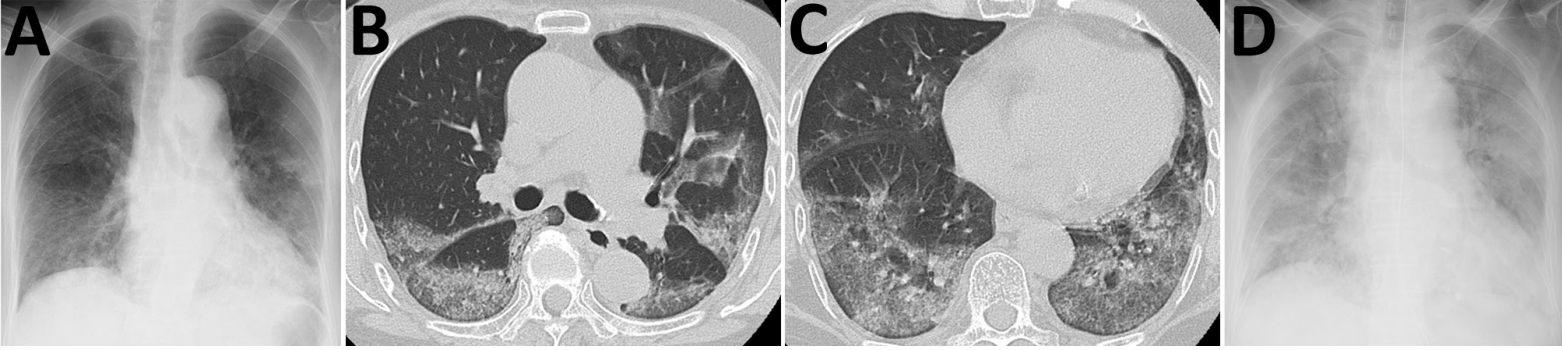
Chest radiograph and computed tomography results from an 84-year-old woman who died from coronavirus disease, Toshima Hospital, Tokyo, Japan, February 2020. A) Chest radiographs taken on admission (illness day 8), showing reticular shadows, mainly in bilateral lower lung fields. B, C) Chest computed tomography scan taken on illness day 8, indicating ground-glass opacities mainly located in posterior segments of the bilateral lower lobes, where the highest numbers of viral RNA copies were found on autopsy. D) Chest radiographs taken on illness day 14, with shadows spreading to almost entire lungs and exhibiting air bronchograms.
